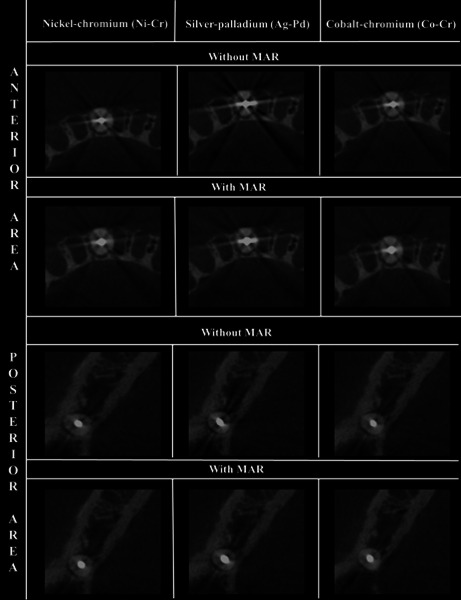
Cropped CBCT reconstructions (axial view) of each metal post
